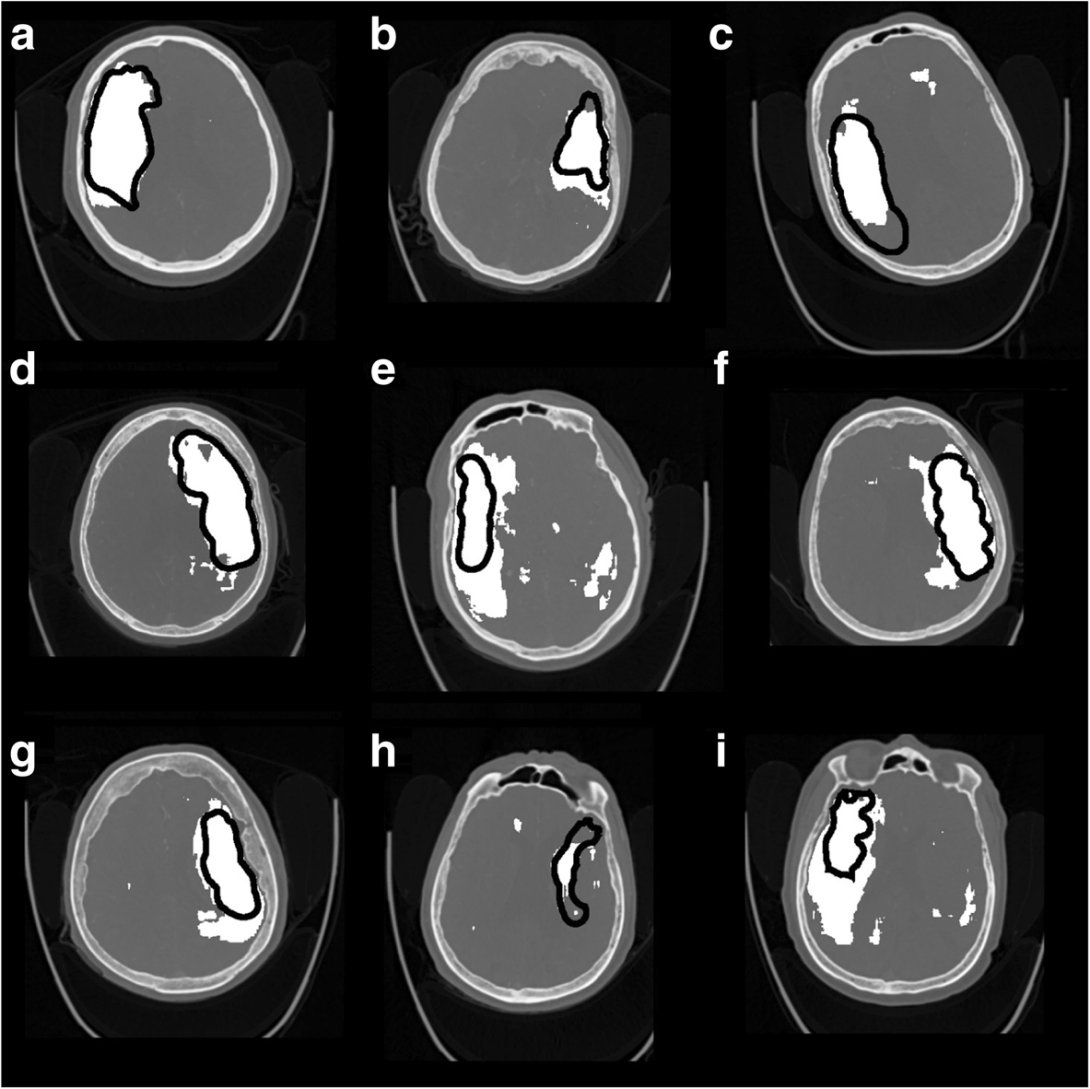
Representative slices of nine (a-i) of the infarct positive test cases. 3D CNN output with CTA + cerebral hemispheric comparison + NCCT features are shown in white, and the infarcted lesion drawn manually is indicated with black perimeters
